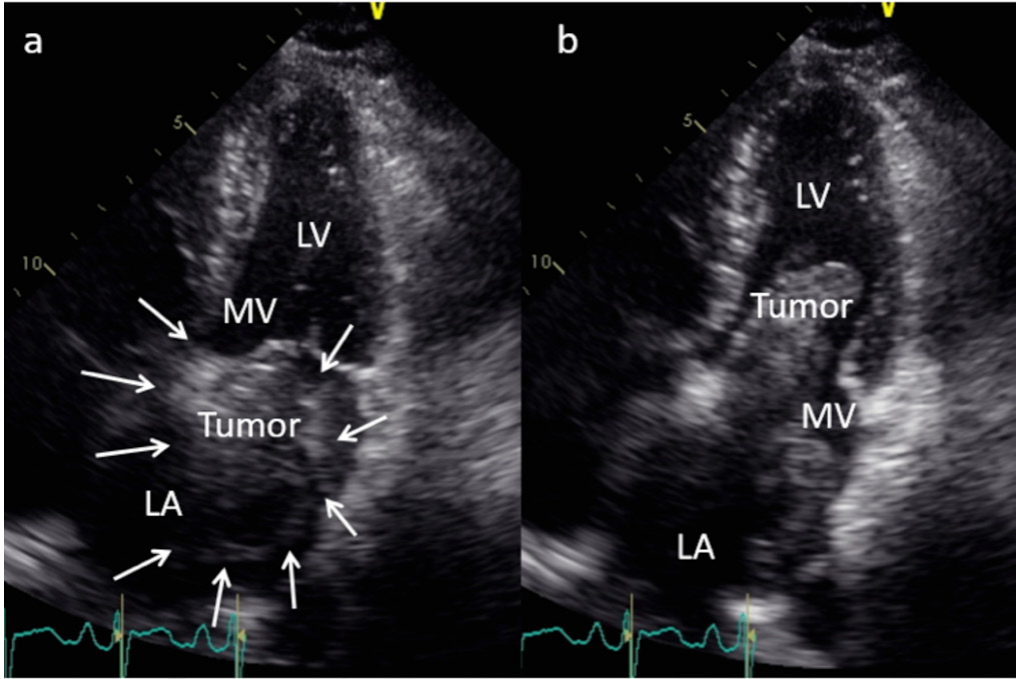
A) Echocardiography showed a well demarcated huge mass in the left atrium (arrows); B) Left atrial tumor herniated to the mitral valve orifice; LV=left ventricle; MV=mitral valve; LA=left atrium
